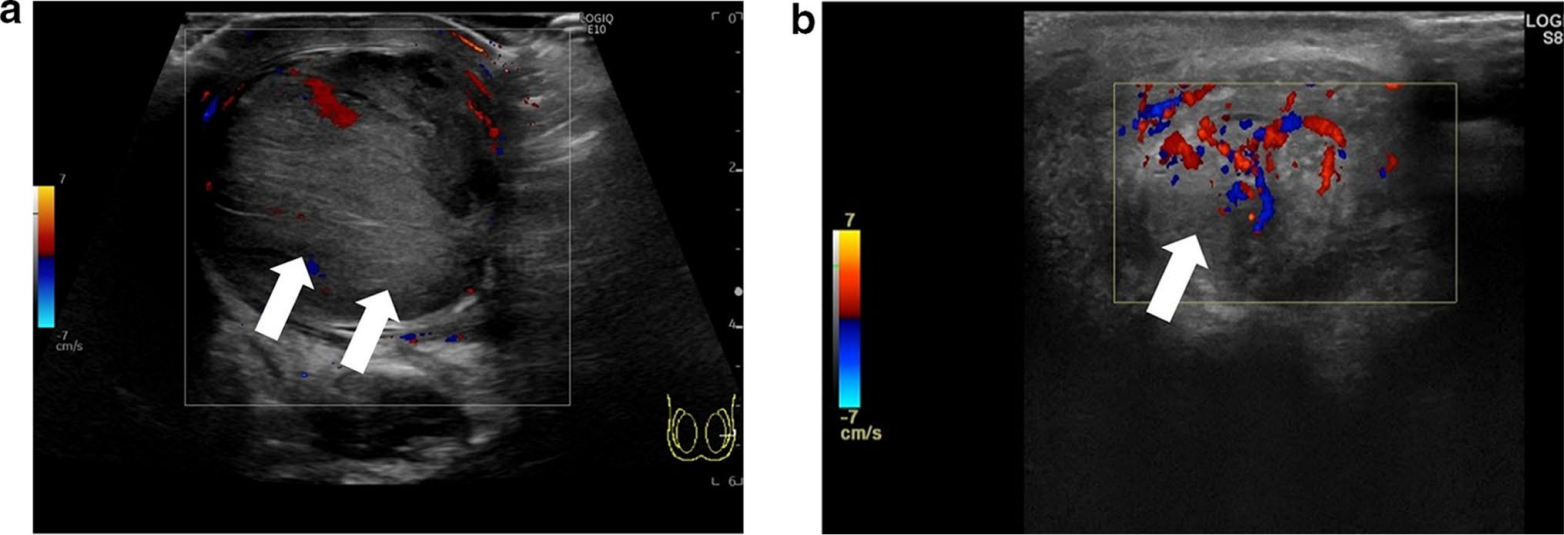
Ultrasonographic images of a 13-year-old boy with epididymitis accompanying segmental testicular infarction. a The upper pore of the right testis shows heterogeneous echogenicity. b The epididymis shows hypervascularity; therefore, epididymitis accompanying segmental testicular infarction was diagnosed
